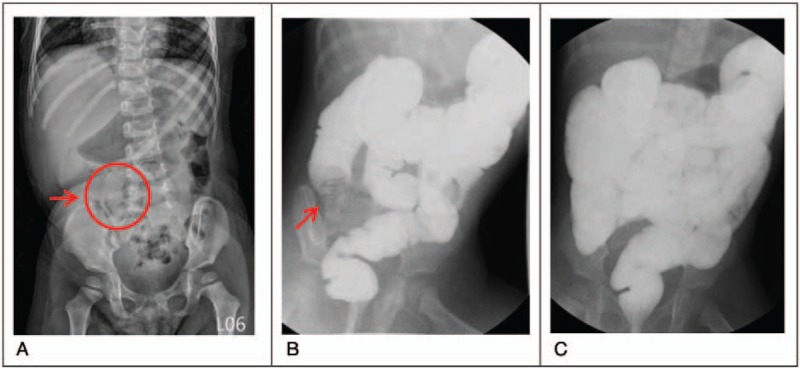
Twin A. A, Abdominal plain film revealing a mass lesion at the right upper quadrant (red arrow). B and C, Lower gastrointestinal series revealed ileocolic intussusception (red arrow) and successful reduction.
